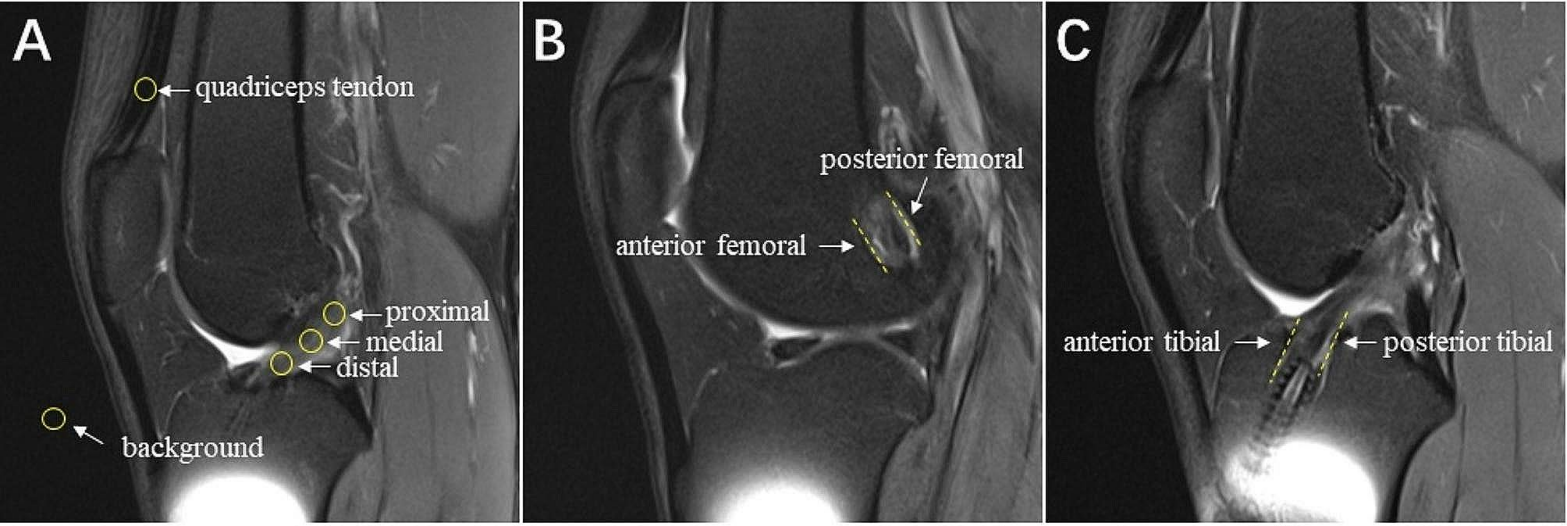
The evaluation of autograft maturation and graft-bone interface healing on MRI images. A. The SI of hamstring autograft (yellow circle) was obtained on the proximal, medial and distal segments of graft. SNQ = (SI of hamstring autograft - SI of quadriceps tendon) / SI of background. B and C. The anterior and posterior SI of graft-bone interface at femoral tunnel and 1/3 proximal tibial tunnel (yellow imaginary line) was obtained and classified into 4 grades. SI: signal intensity, SNQ: signal/noise quotient
